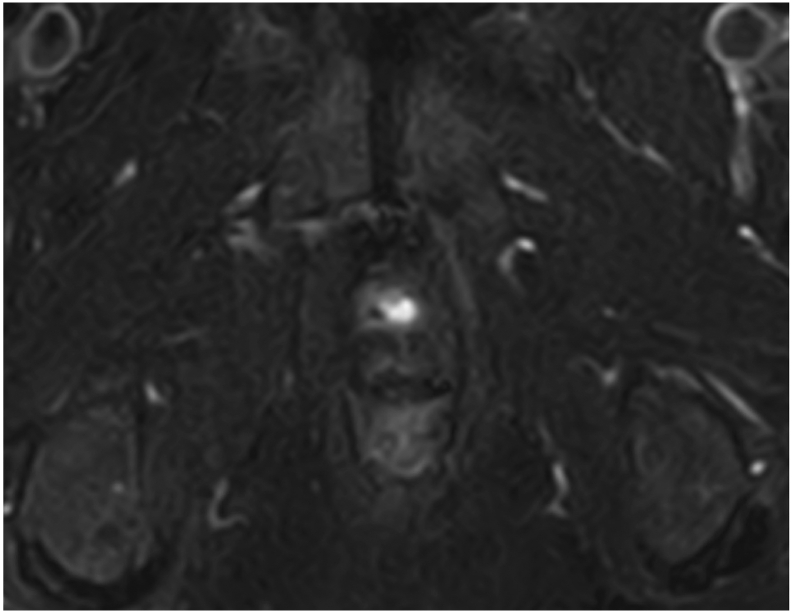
MRI at 6 months after closure of the fistula showed that the fistula had disappeared.
